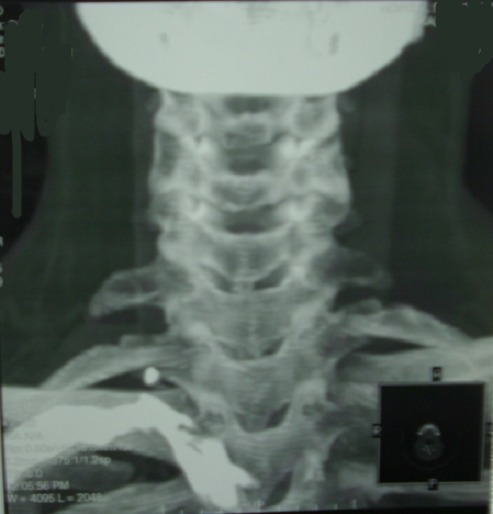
Radiographies de face objectivant la côte surnuméraire chez une patiente de 24 ans
